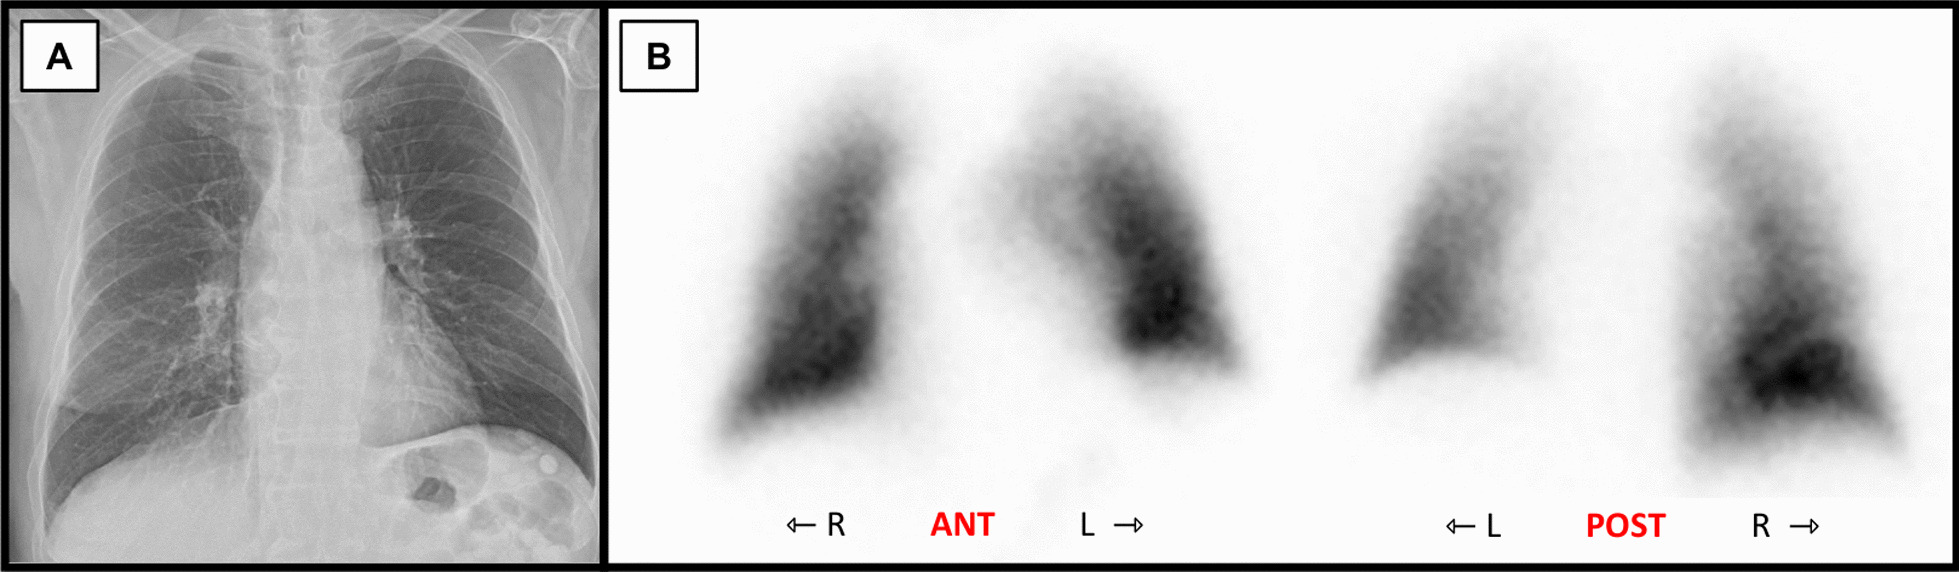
A Postoperative x-ray without signs of pulmonary congestion. B A V/Q scan on the 29th postoperative day showed symmetrical perfusion of the donor lung
